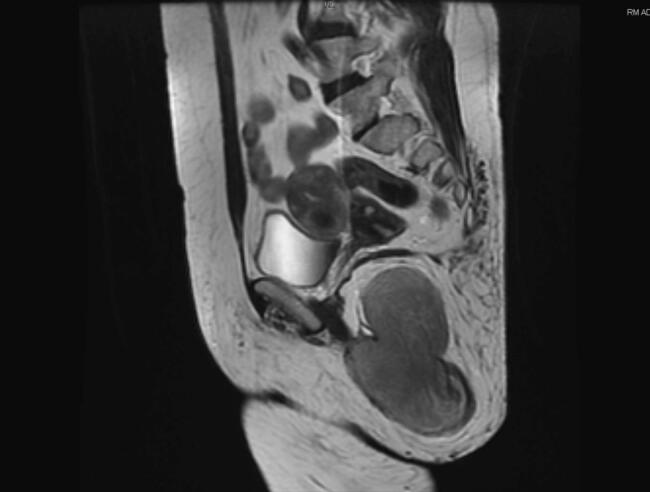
MRI shows a lump in the right ischiorectal fossa after contrast enhancement.
